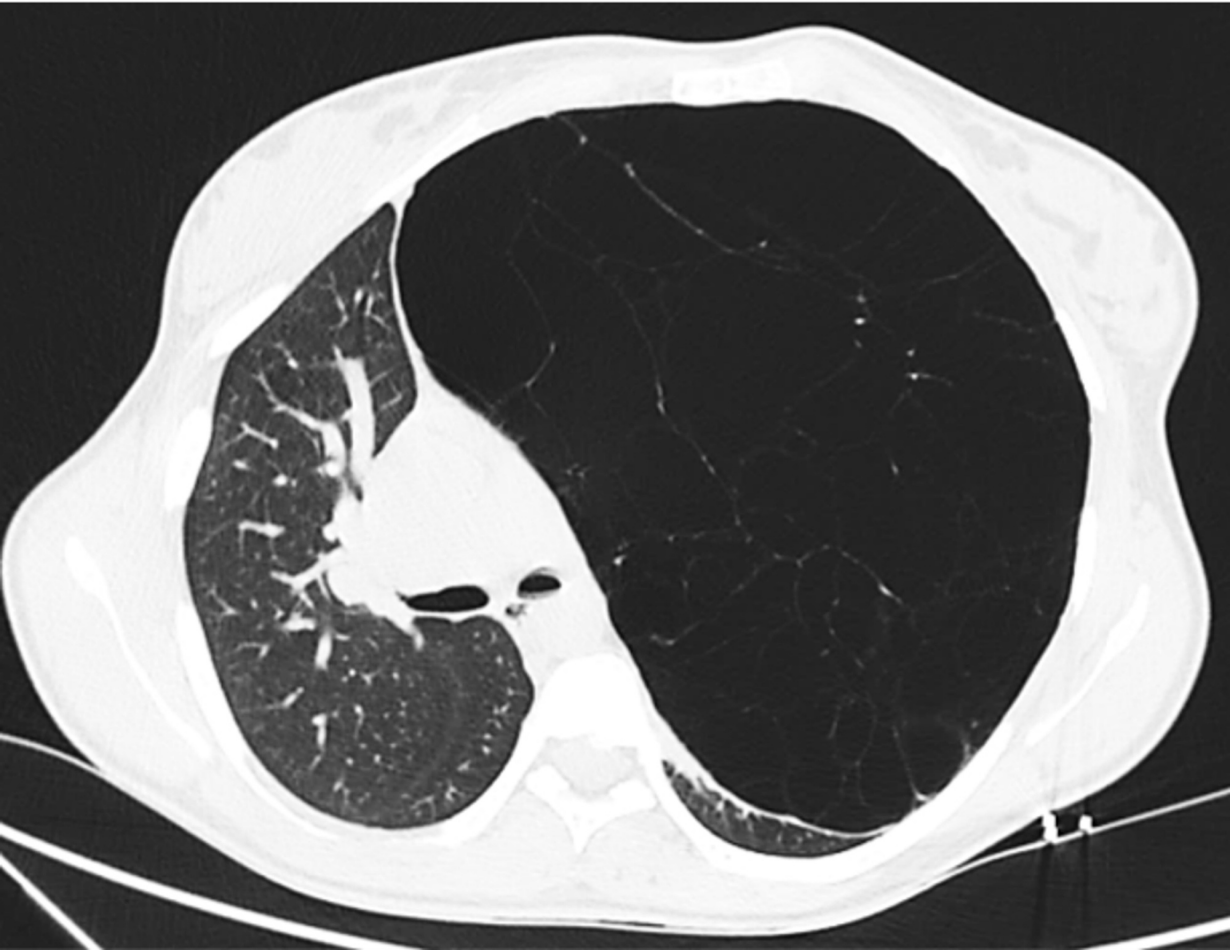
Chest computed tomography (axial).
